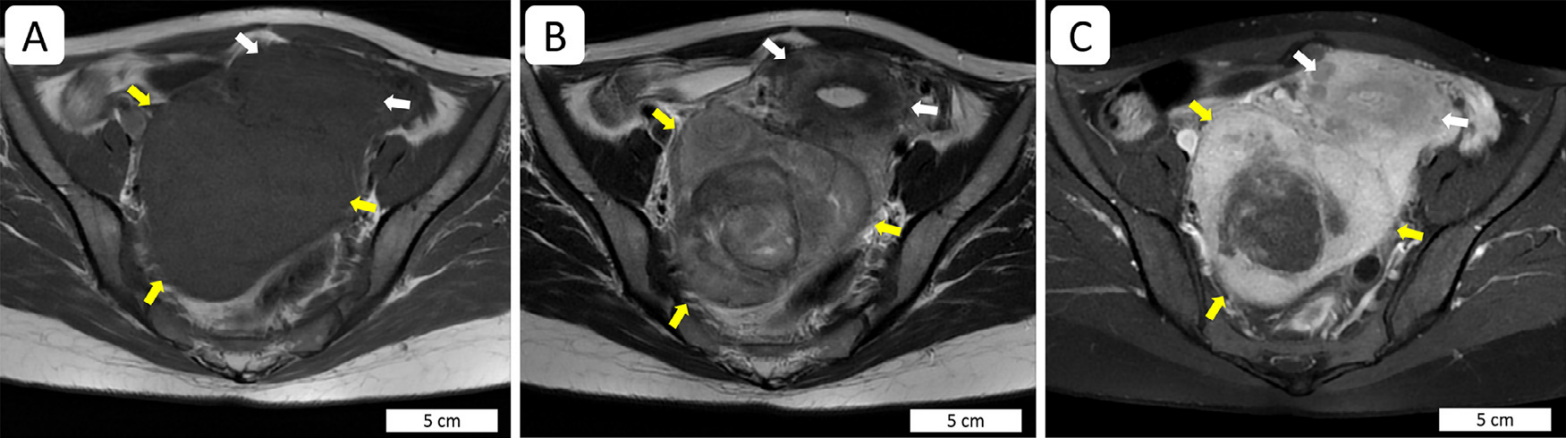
Contrast-enhanced, axial, magnetic resonance images of a schwannoma-like uterine leiomyoma. A schwannoma-like leiomyoma (yellow arrows) is located at the posterior aspect of the uterus (white arrows). The tumor shows low intensity on a T1-weighted image (A) and high intensity on a T2-weighted image (B). The contrast-enhanced image (C) demonstrates a nonenhanced lesion in the tumor. Scale bars are shown in the images.
